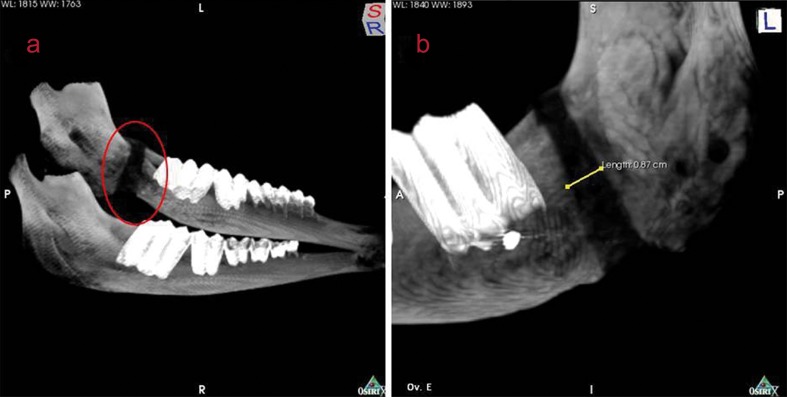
A) Three-dimensional, maximal intensity projection reconstructions of computed tomography images and B) linear measure between the edges of mature bone.
